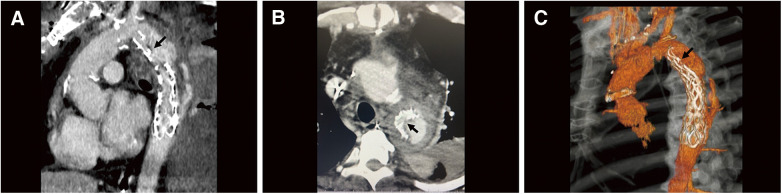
Fig. 2 Enhanced CT imaging (A, B, C) 2 months after surgery shows flattening of the FET as a consequence of the persistent endoleak (black arrow). CT: computed tomography; FET: frozen elephant trunk
